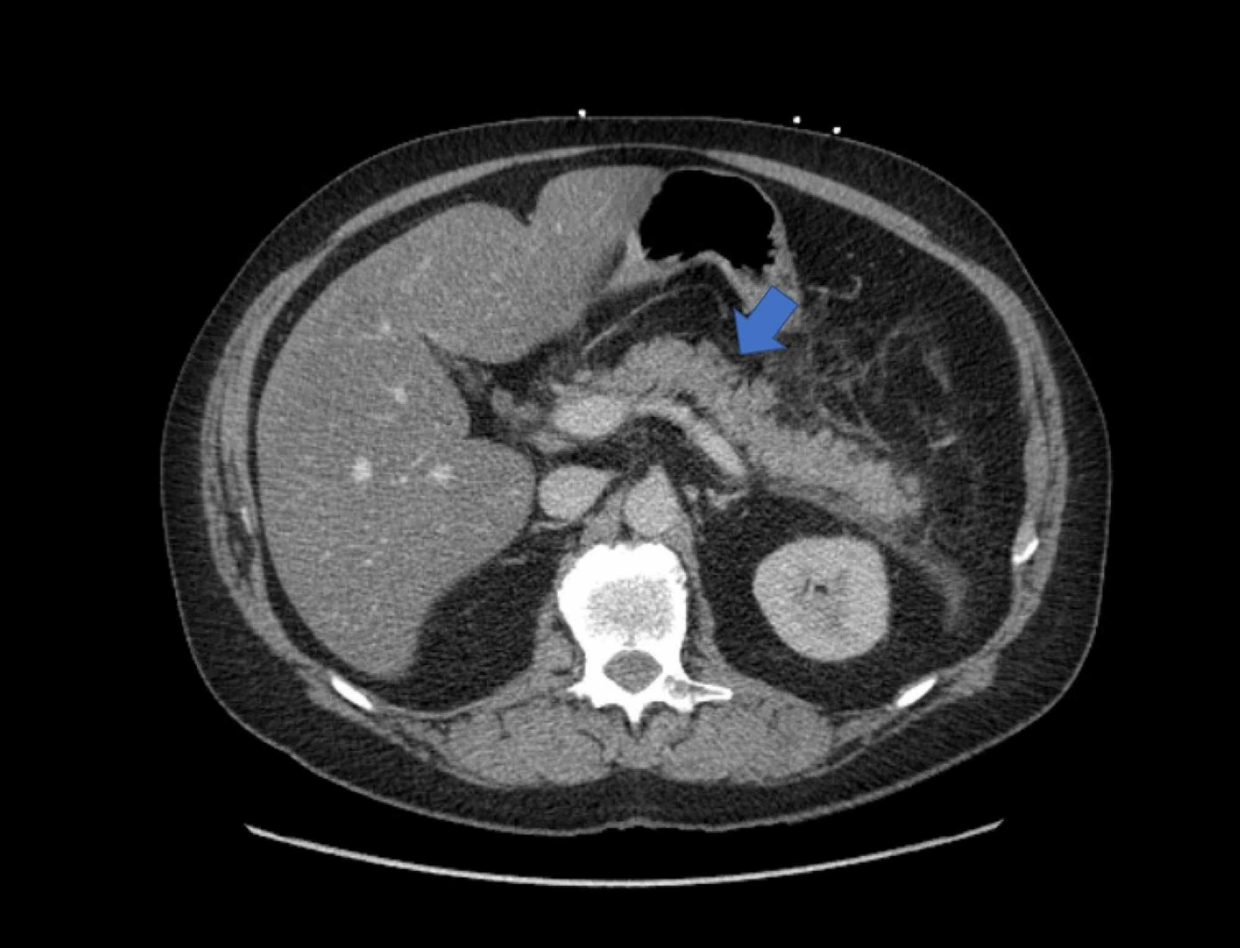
CT scan of abdomen demonstrating acute interstitial edematous pancreatitis.
